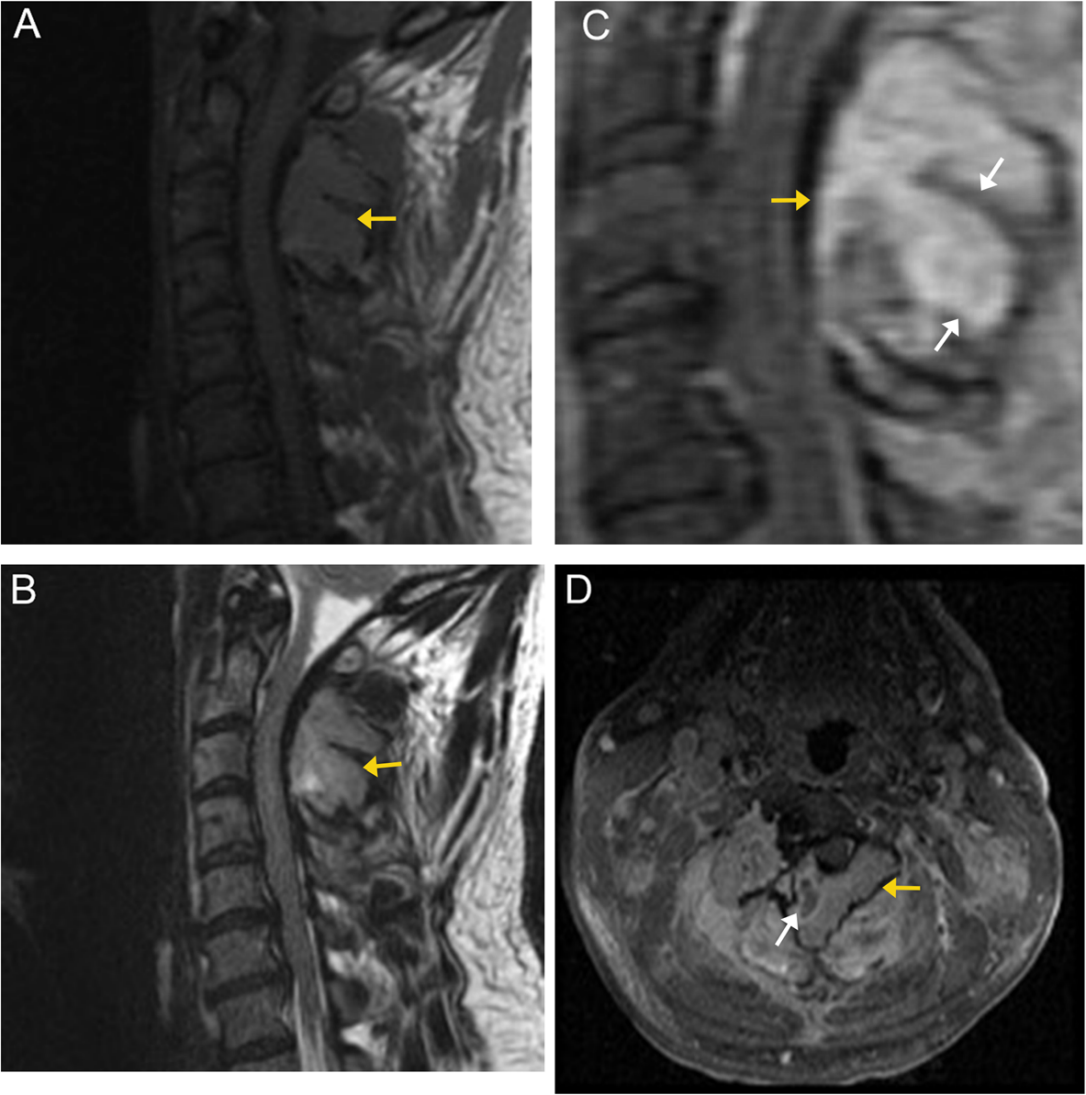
Plasmacytoma. Sagittal and axial MR images demonstrate an expansile mass (orange arrows) arising from the posterior elements of C2 and C3. The mass demonstrates hypointense signal to normal marrow on T1 (a), and isointense to hyperintense signal on T2 (b). Post-contrast sagittal T1 (c) and axial T1 fat-suppressed (d) sequences demonstrate enhancement. A few areas of curvilinear low-signal intensity are seen at the remaining thickened bony cortex (white arrows), projecting into the lesion, resulting in a “mini-brain” appearance resembling cortical sulci
